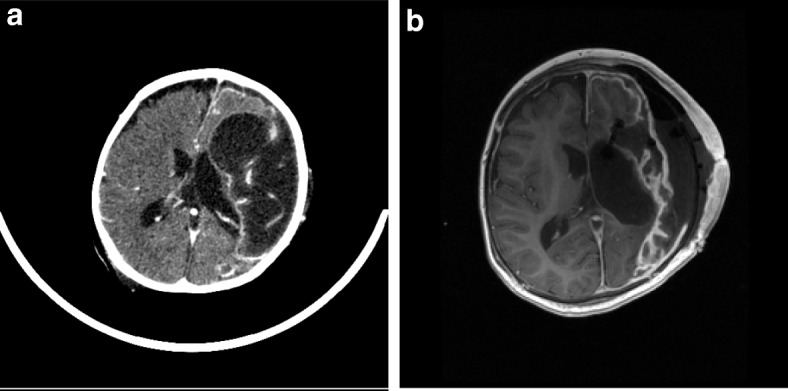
a: Axial contrast-enhanced CT day 10 of illness reveals an extensive area of cystic encephalomalacia in the left middle cerebral artery territory with marginal enhancement. Pial enhancement is evident of the entire left cerebral hemisphere. There is longstanding ex vacuo dilatation of the left lateral ventricle and new mass effect with shift of the anterior falx to the right. b: Axial contrast-enhanced T1 MRI on day 21 after two neurosurgical interventions. The extensive left craniotomy, evacuation and debridement of the infected encephalomalacic tissue is evident with pronounced pial and leptomeningeal enhancement of the left cerebral hemisphere. There is discontinuity of the dura with overlying extradural fluid and air. An extra-ventricular drain tip is located in the anterior left lateral ventricle
